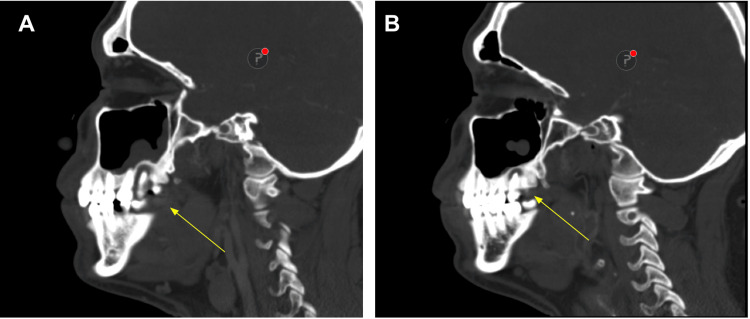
CT of the maxillofacial area with and without IV contrast CT of the maxillofacial area with and without IV contrast showing (A) right side of the face and (B) left side of the face. Cavities and periapical abscesses are noted involving the maxillary and mandibular teeth, more prominent on the right side than the left side. Mucosal thickening of the biliary maxillary sinus is seen on the right side more than the left side. CT, computed tomography
